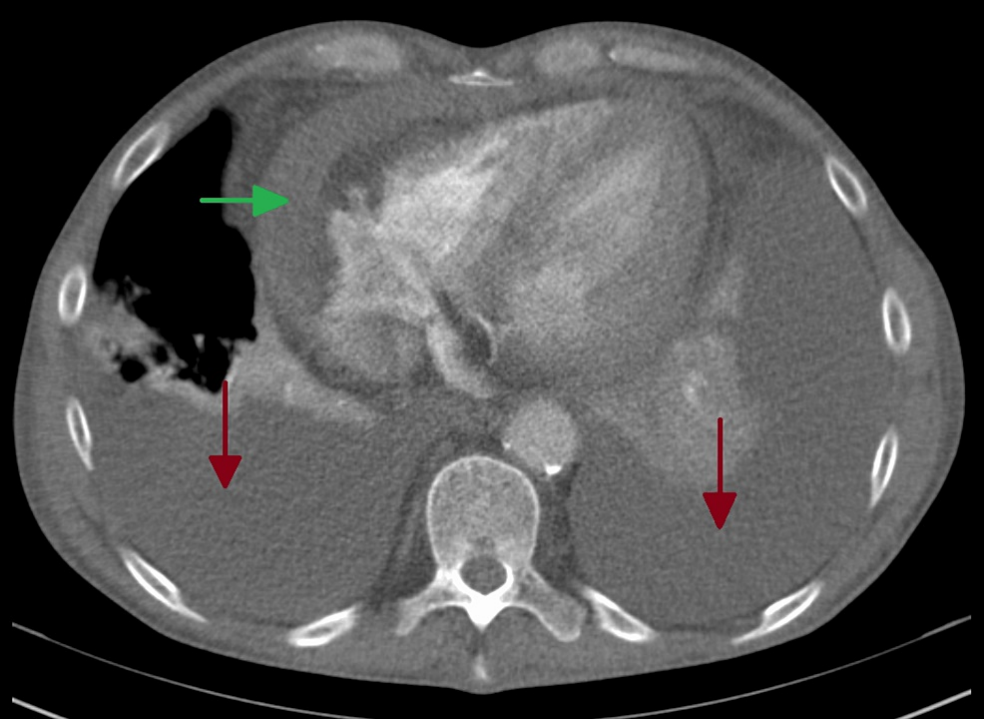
Chest computed tomography Red arrows: bilateral pleural effusion; green arrow: pericardial effusion
